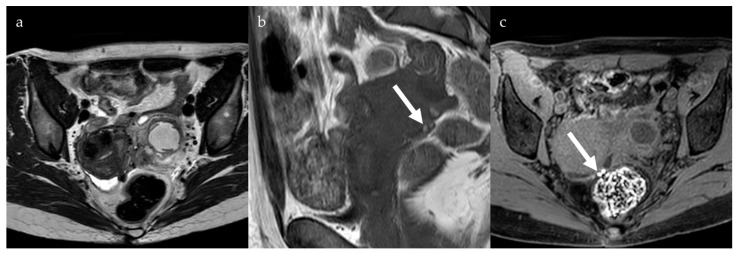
Hypointense nodules in the pouch of Douglas on an axial T2-weighted image (a) with some small high-signal foci on a coronal T1-weighted image (b, arrow), more evident on an axial fat-saturated T1-weighted image (c, arrow), compatible with deep pelvic endometriosis with evidence of bleeding.
